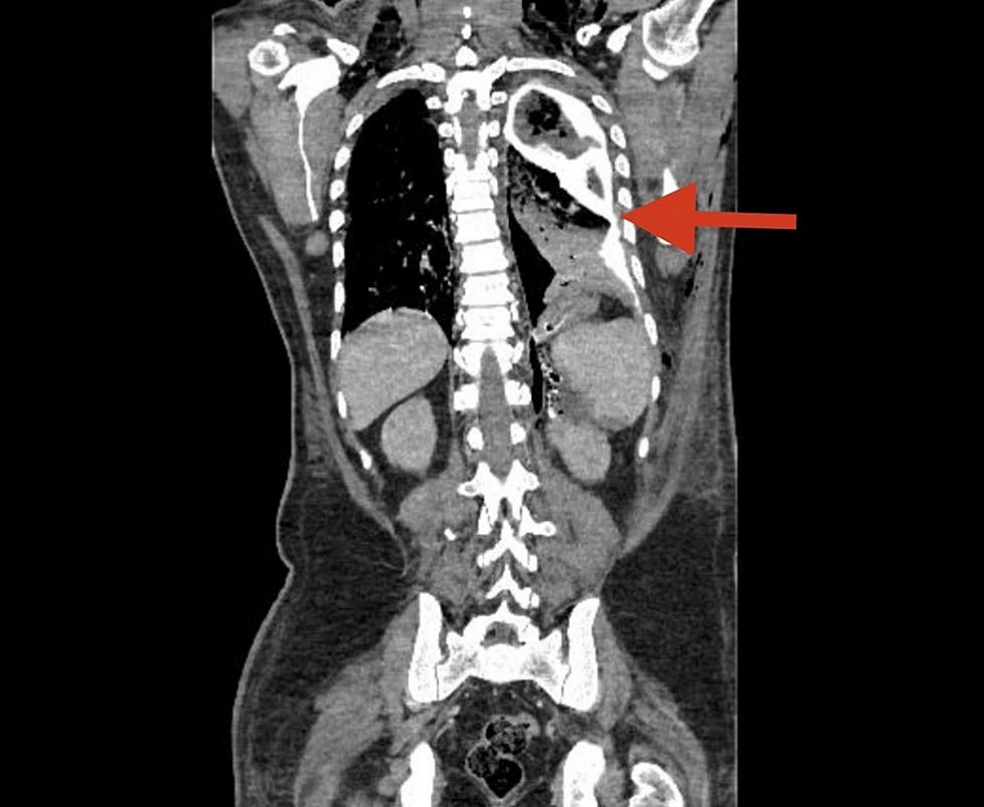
A coronal section of contrast-enhanced CT showing oral contrast extravasation (red arrow) into the left pleural space, indicating a fistulous opening from the GI tract into the pleural space.
